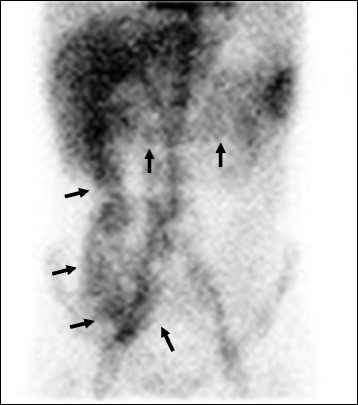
^99m^Technetium-human serum albumin-diethylenetriaminepenta-acetic acid scintigraphy demonstrated that the extravasation of radioactive isotope was detected at terminal ileum, cecum, ascending and transverse colon (arrow), but not at the oral side of terminal ileum
